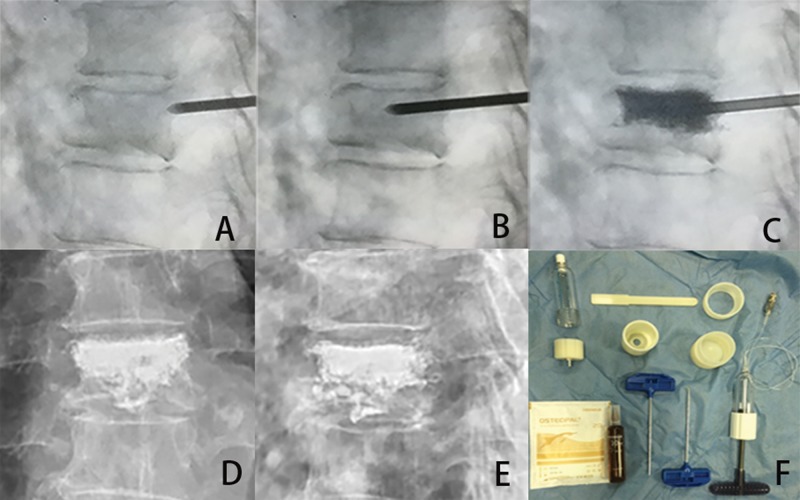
HPDS surgical procedure for the treatment of a 79-year-old female patient with T8 vertebral body fracture. A, A puncture needle enters into the fractured T8 vertebra via the left pedicle. B, Lateral radiograph showing the puncture needle tip in the anterior column of the vertebral body. C, High-viscosity bone cement is injected into the fractured vertebral body. The lateral radiograph shows the filling of the vertebral body with high-viscosity cement. D and E, Lateral and posterior-anterior X-ray films after the HPDS surgical procedure. F, The HPDS device and high-viscosity cement.
